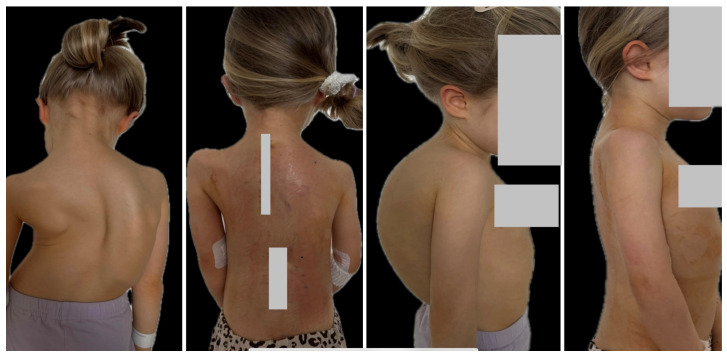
The X-rays and clinical images of a 4-year-old girl with severe early onset idiopathic scoliosis, who underwent pre-operative HGT and surgical proceeding with minimally invasive controlled growing rods placement [46].
